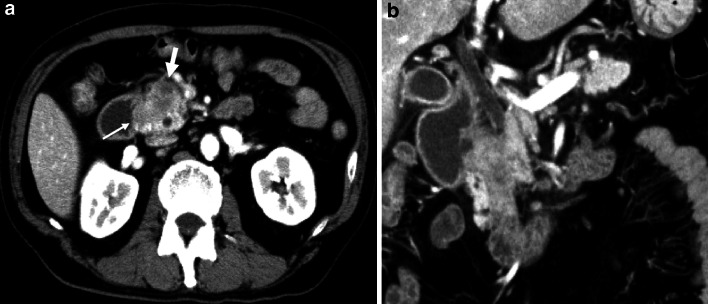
a Enhanced CT shows pancreatic parenchyma encircling the descending part of the duodenum (*thin arrow*); and b low density lesion is observed in the annular pancreas (*thick arrow*)
